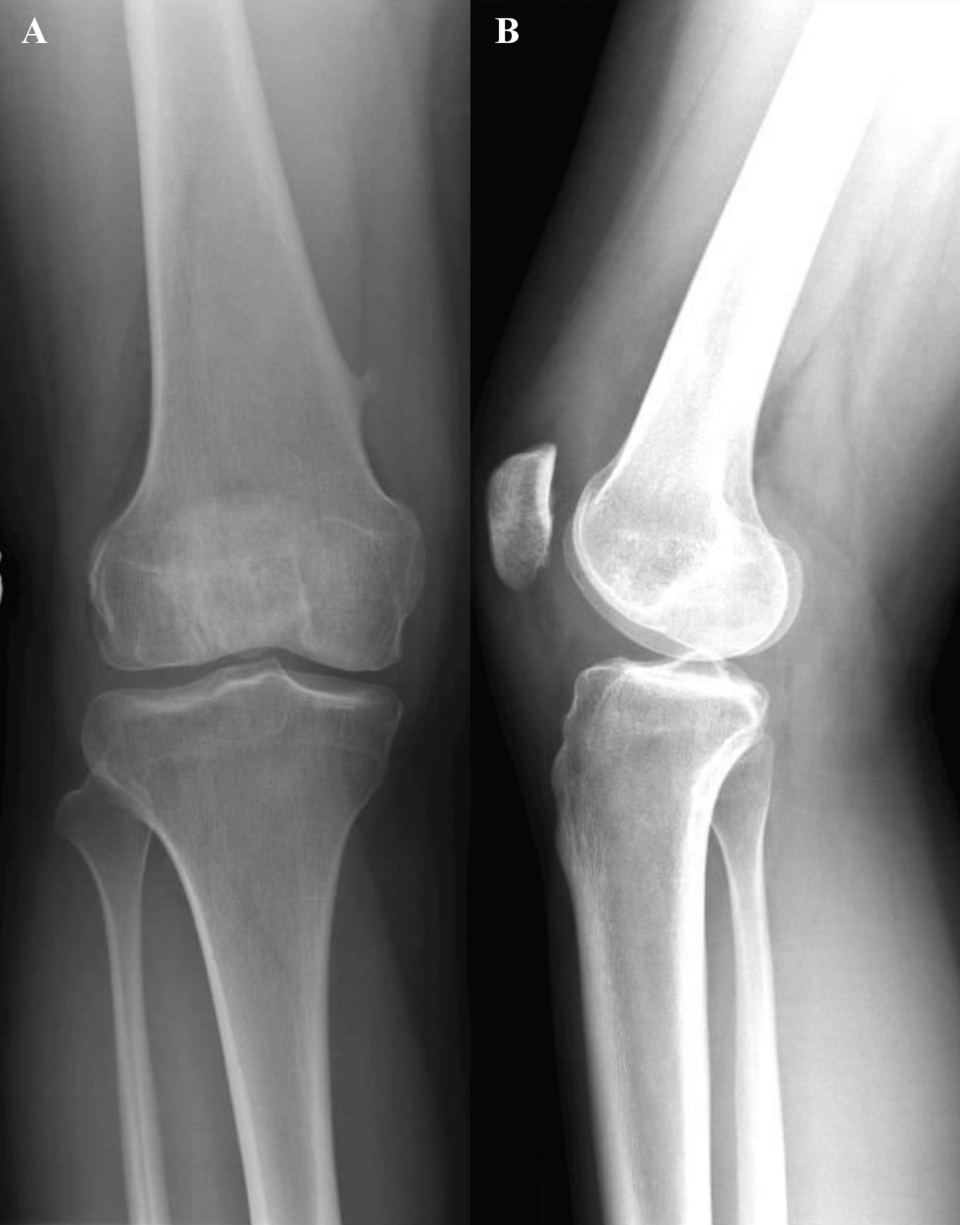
Eight-month post-operative X-rays demonstrate removal of the osteochondral defect from the inferior aspect of the medial patellar facet, with coronal view (A) showing re-insertion into the posterior lateral femoral condyle and sagittal view (B) depicting the patellofemoral surface post-removal. A: Post-operative coronal X-ray of posterior tibiofemoral joint portraying removal of osteochondral defect from inferior aspect of medial patellar facet and re-insertion into posterior lateral femoral condyle. B: Post-operative sagittal X-ray of patellofemoral surface portraying removal of osteochondral defect from inferior aspect of medial patellar facet.
